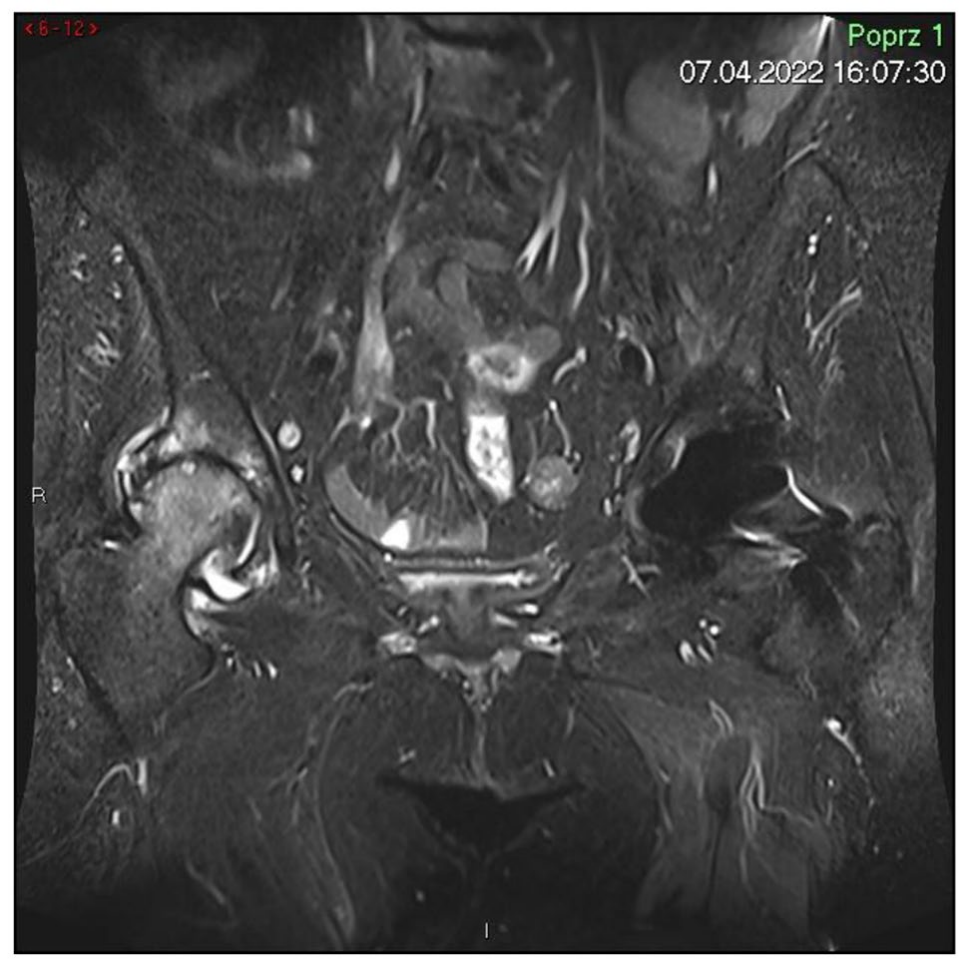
Last MRI scan with full hematoma regression
